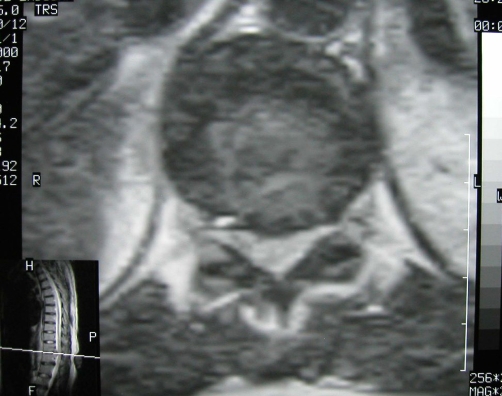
Magnetic resonance of the thoracic spine at T11-12 level (T2-weighted, axial) shows herniated nucleus pulposus and spinal cord compression.
